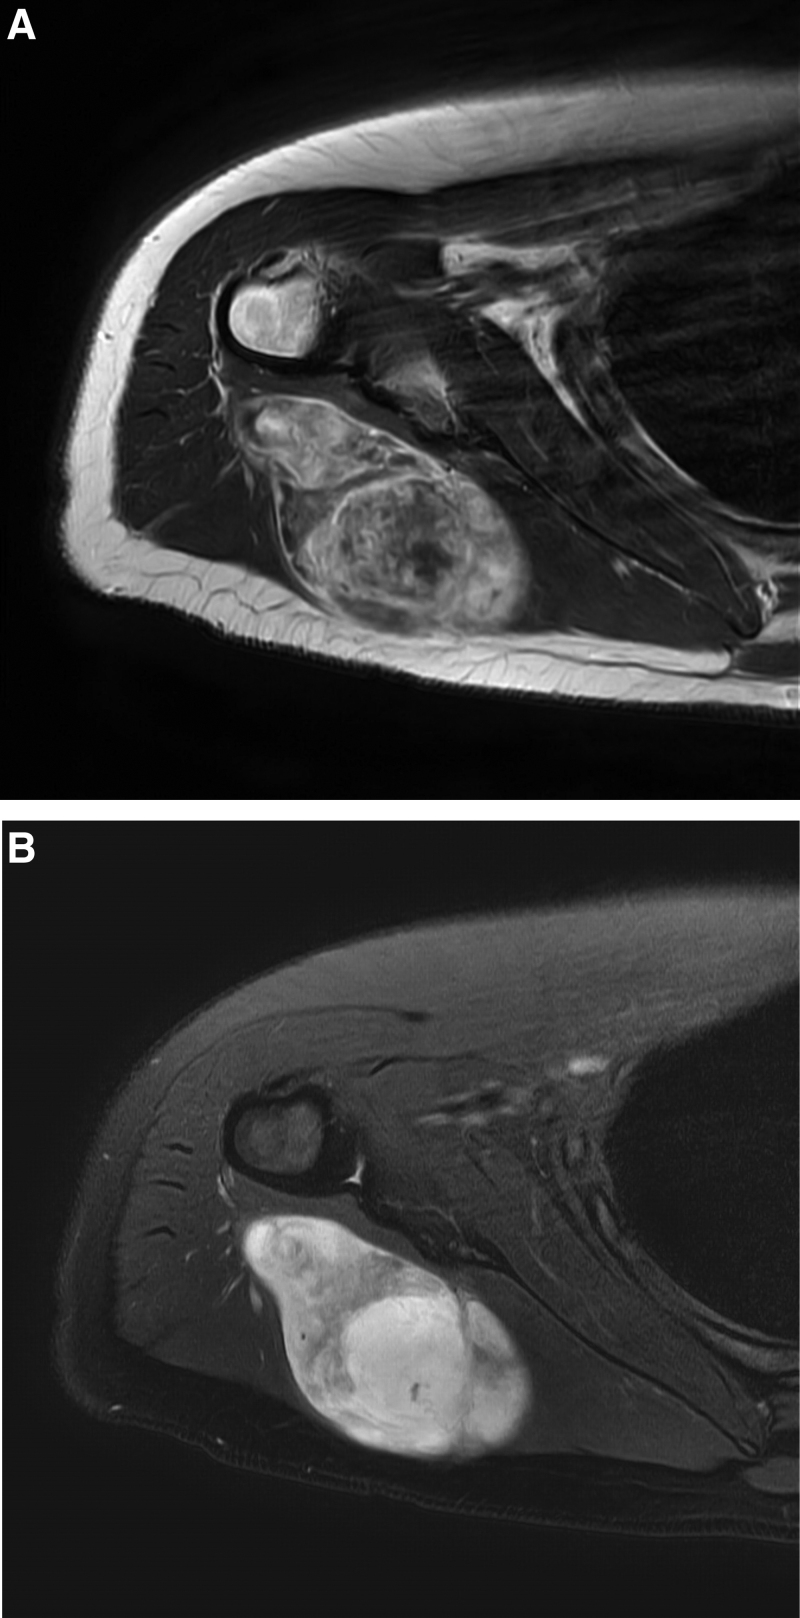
Magnetic resonance imaging showing a 9.0 × 7.0 × 4.0 cm heterogeneous soft tissue mass with a myxoid component in the teres minor muscle. (A) Contrast-enhanced *T1*-weighted imaging. (B) Fat-suppressed *T2*-weighted imaging.
